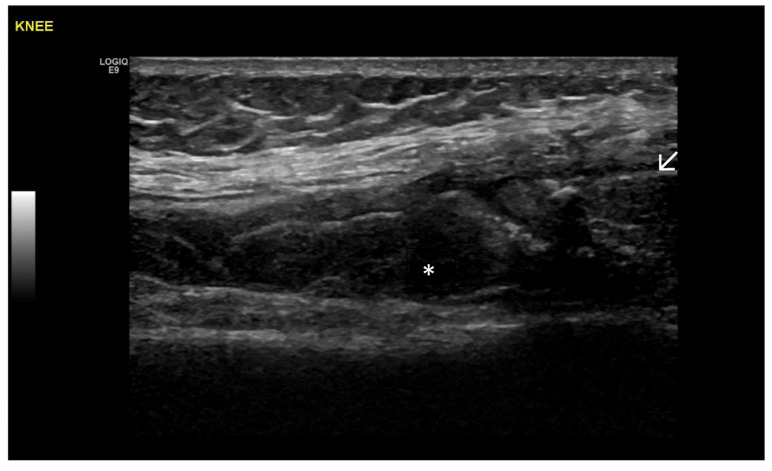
Ultrasonography of the knee joint—longitudinal scan at the level of the suprapatellar recess. Suprapatellar fad pad—arrow. There is pronounced synovial proliferation (asterisk) at the level of the suprapatellar recess, which presents as abnormal hypoechoic intra-articular tissue.
